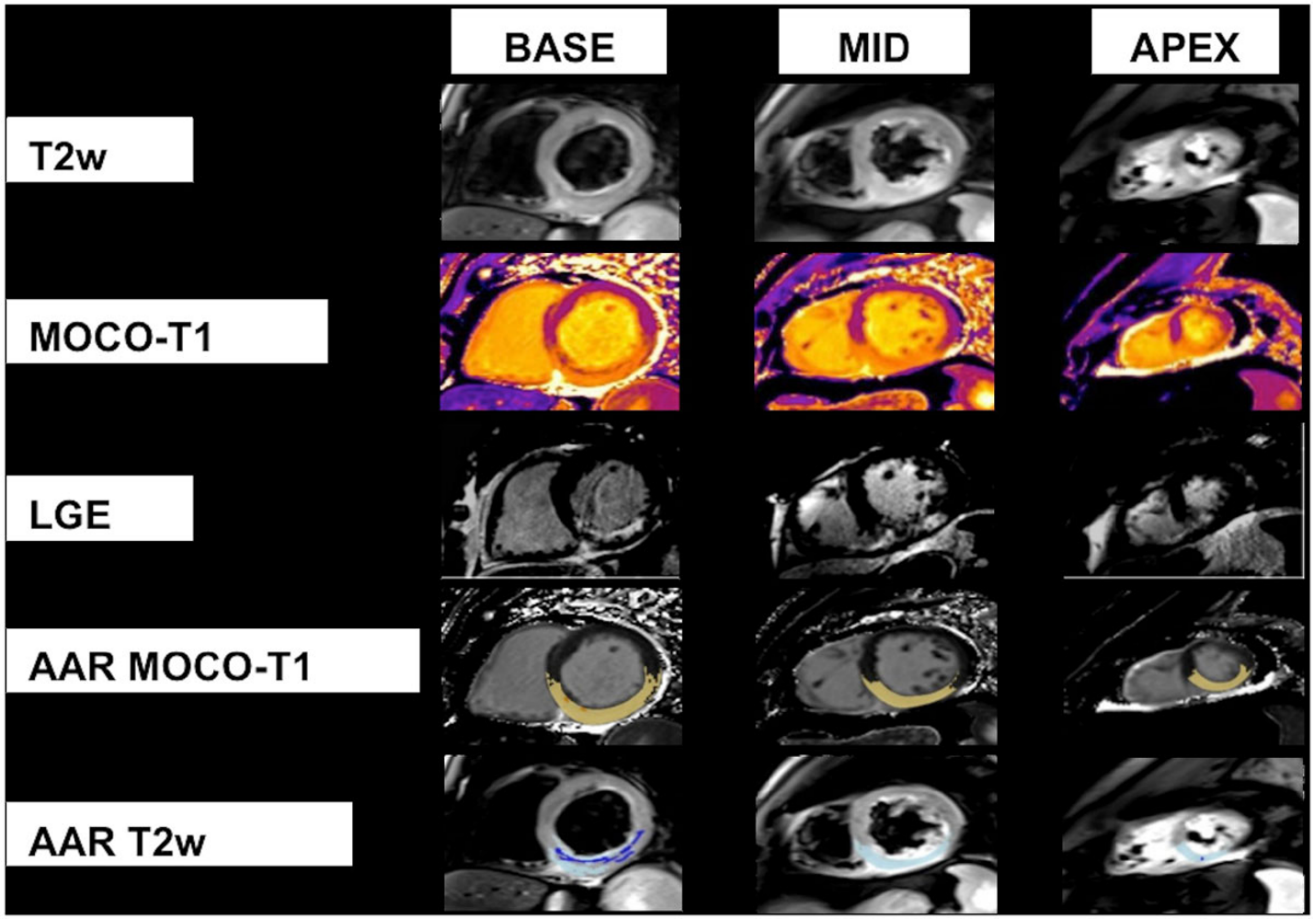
CMR short axis LV images demonstrating enhancement consistent with myocardial oedema (AAR) by T2w imaging (rows 1 and 5) and by MOCO-T1 (rows 2 and 4) referenced to infarction (row 3). *AAR, area-at-risk; CMR, cardiovascular magnetic resonance; LGE, late-gadolinium enhancement; LV, left ventricular; MOCO, motion-corrected; T2w, T2-weighted*
